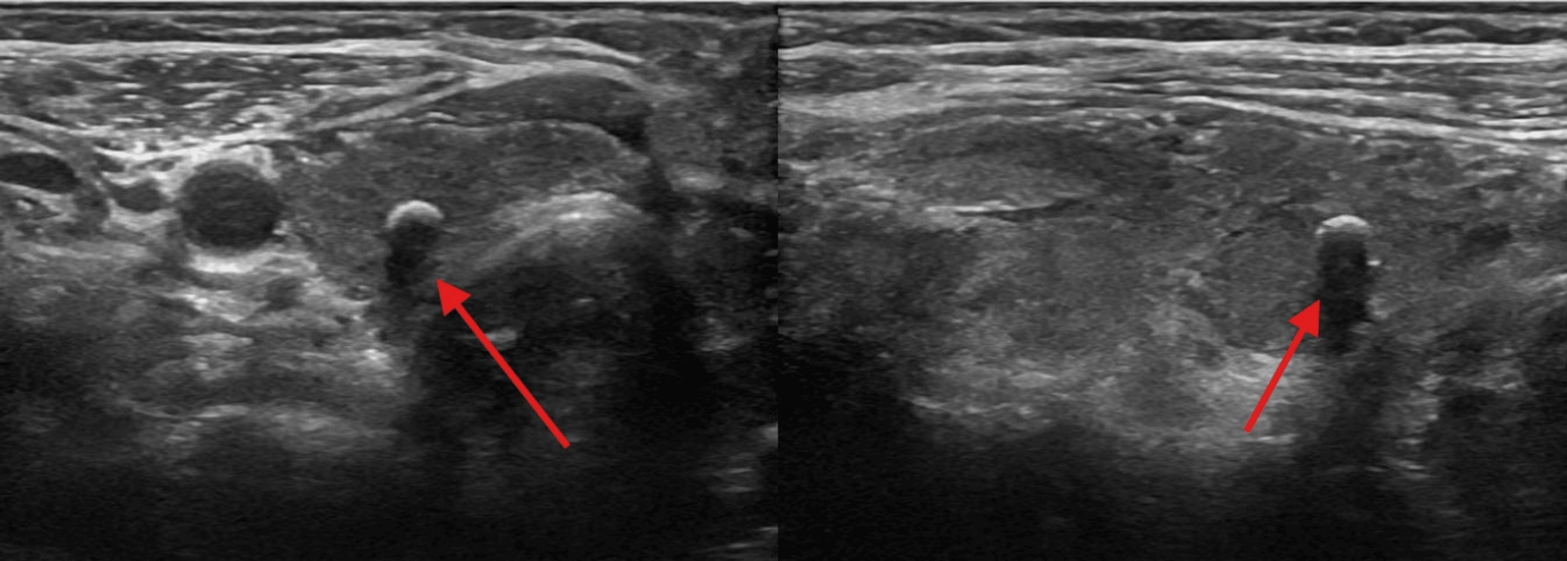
Thyroid ultrasound findings Transverse ultrasound image showing a well-circumscribed nodule in the right thyroid lobe.
